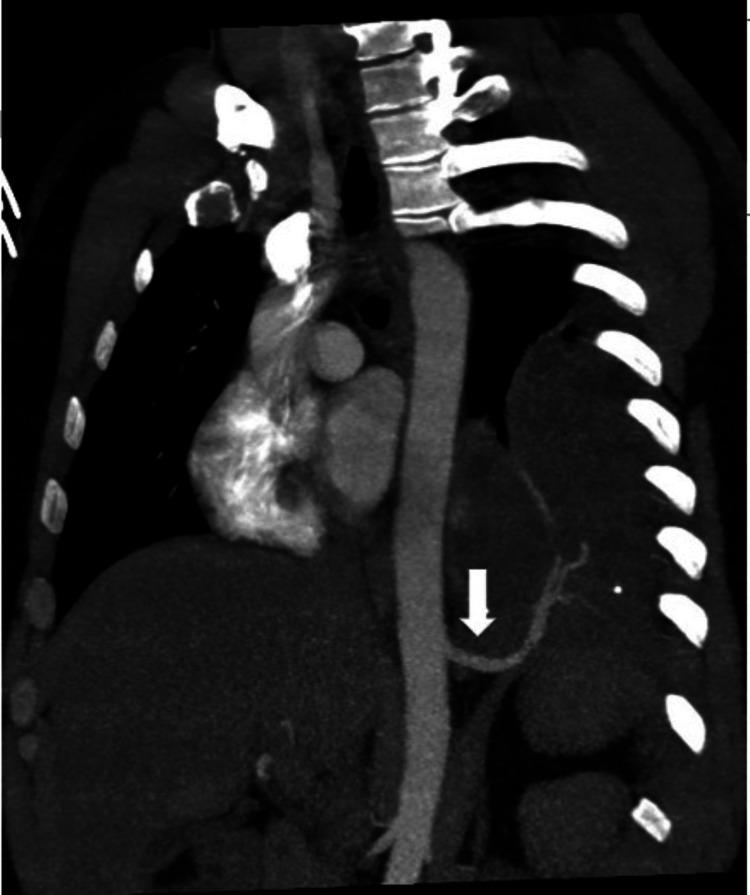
Admission chest CT scan Coronal oblique maximum intensity projection (MIP) reconstructions show an aberrant artery arising from the distal thoracic aorta above the diaphragm. This vessel courses cephalad to supple the sequestration.
